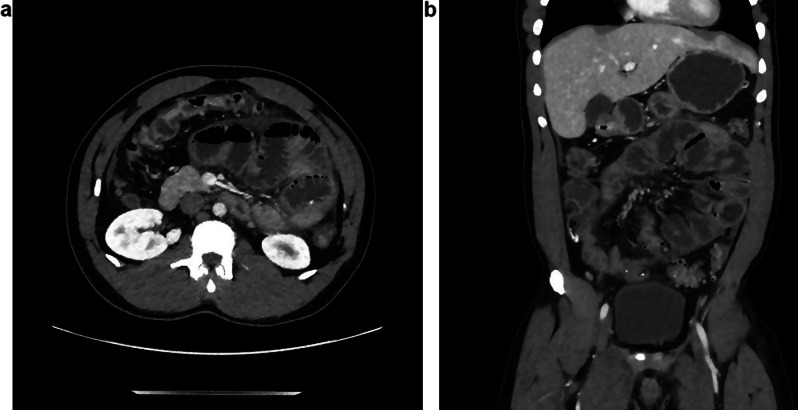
(a) and (b): CT scan 4 weeks later, showing increased intestinal loop distension but less free fluid
